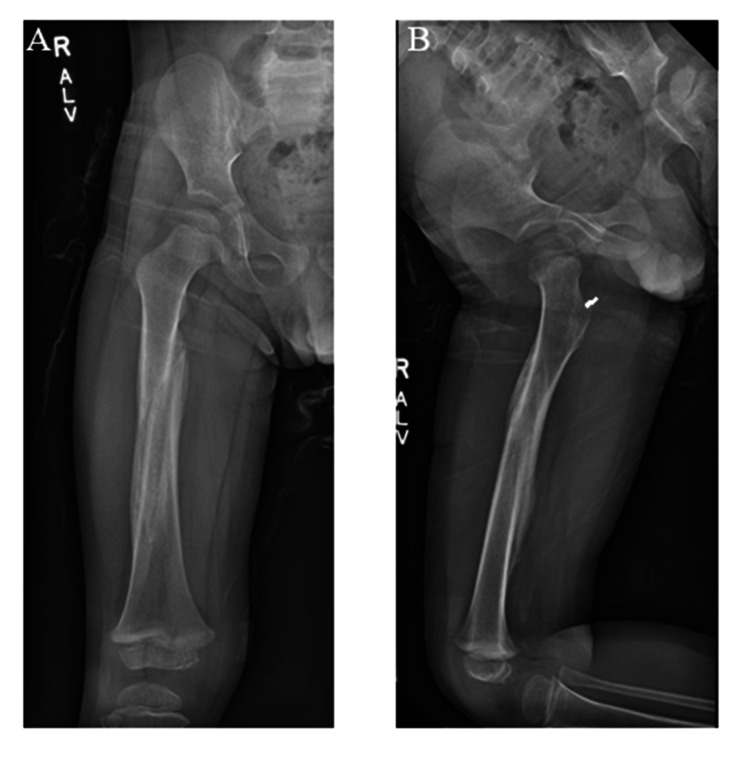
Final imaging for case 3, including (A) AP and (B) lateral radiographs of the right lower extremity demonstrating the healing of oblique subtrochanteric femoral shaft fracture
